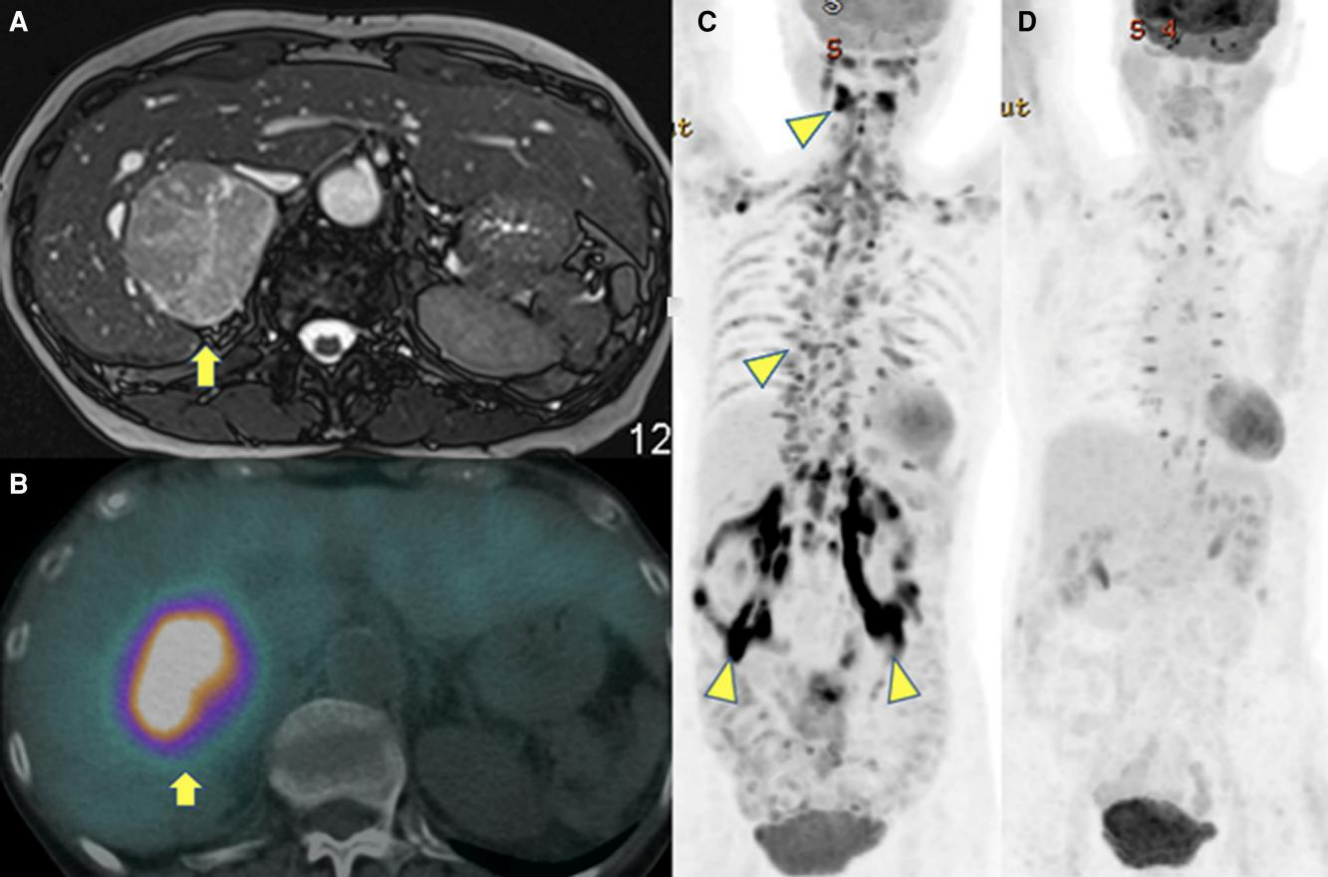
(A) MRI and (B) MIBG imaging demonstrating 7cm MIBG-avid right pheochromocytoma. (C) Pre-operative and (D) postoperative 18-F-FDG-PET/CT imaging demonstrating brown fat activation which resolved following resection of the tumour.
